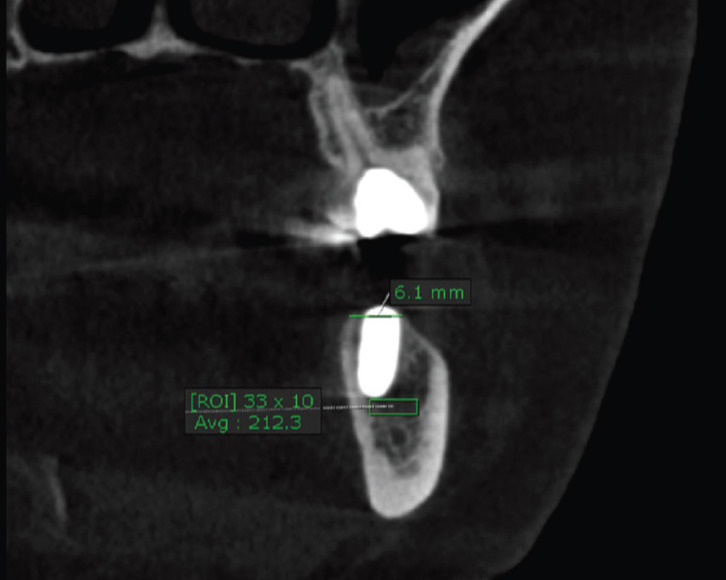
Postoperative coronal view of CBCT showing the average bone density of the same area shown in figure 1 apical to the implant length in the early postoperative period (212.3 Hounsfield unit).
